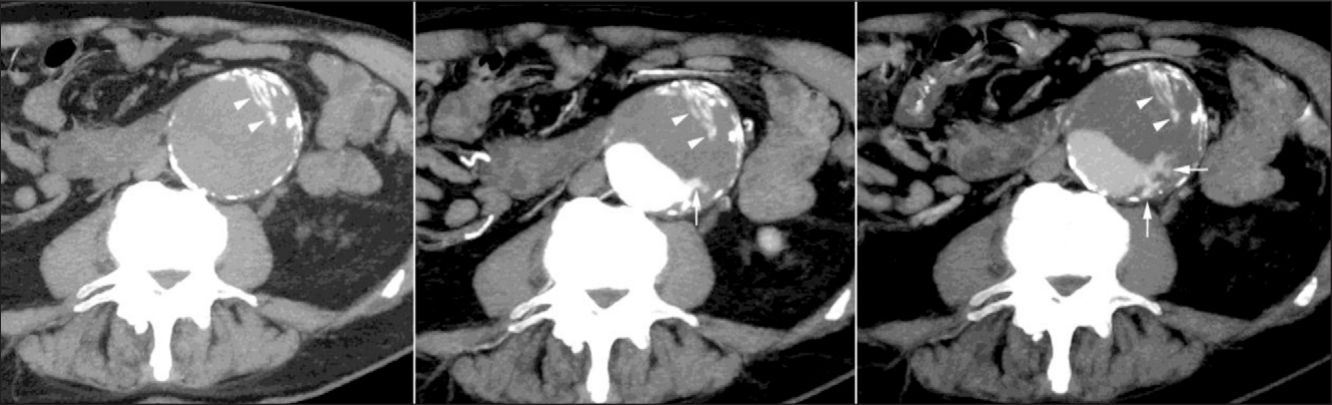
Transverse maximum intensity projection thick-slabs CT of a large AAA obtained before (left panel) and on arterial (centre panel) and portal (right panel) phases after intravenous injection of iodine contrast agent, showing both calcifications (arrowheads) and progressively enhancing vascular channels inside ILT (arrows).
